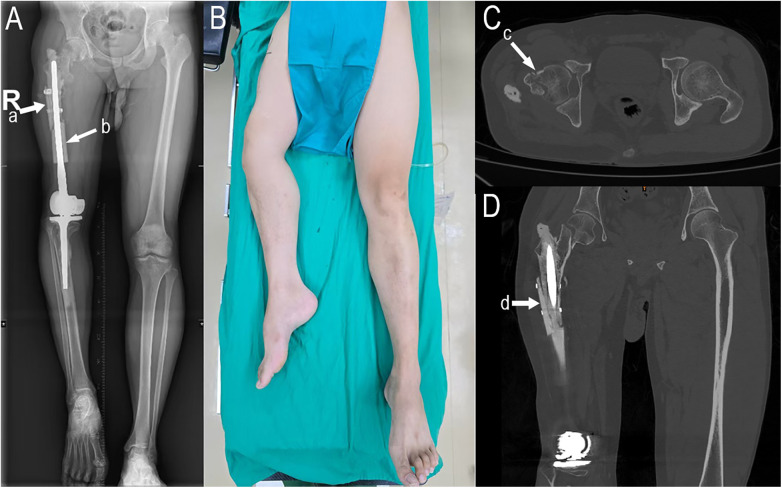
Preoperative radiographic and computed tomography findings. (A) Full-length anteroposterior radiograph of both lower limbs shows the retained femoral tumor endoprosthesis and prior proximal femoral reinforcement; the cerclage wire at the previous fracture site (a) and the homogeneous “artificial bone plate” construct (b) are indicated. (B) Preoperative operating-room photograph demonstrates marked right lower-limb muscle atrophy with limb shortening. (C) Axial CT image demonstrates the femoral neck fracture (c). (D) Coronal CT reconstruction demonstrates the corresponding fracture/reinforcement region (d), consistent with the cerclage-wire location in panel (A) (a) CT = computed tomography.
